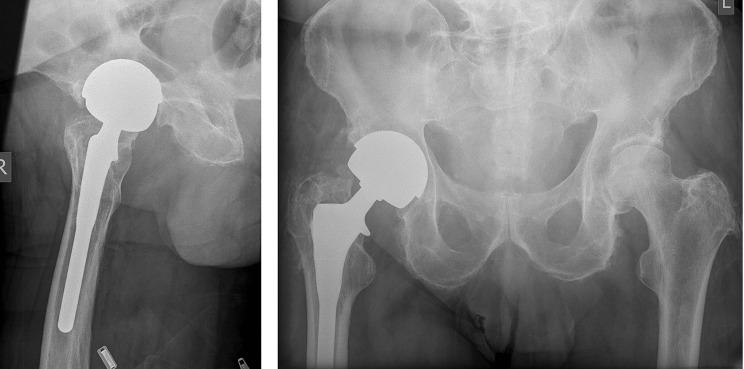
Anteroposterior (AP) and lateral radiograph of the right hip showing a Furlong uncemented metal-on-metal hip replacement with no radiological signs of loosening or osteolysis.
